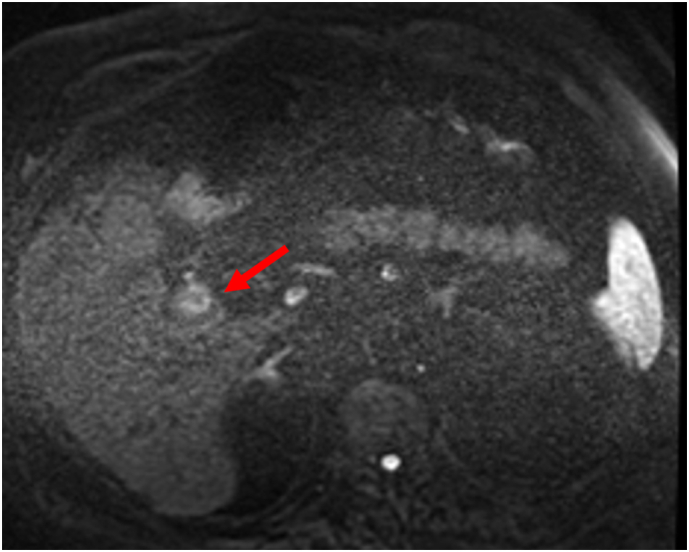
Ax DWI b-1000 shows rim high signal at periphery (arrow).
